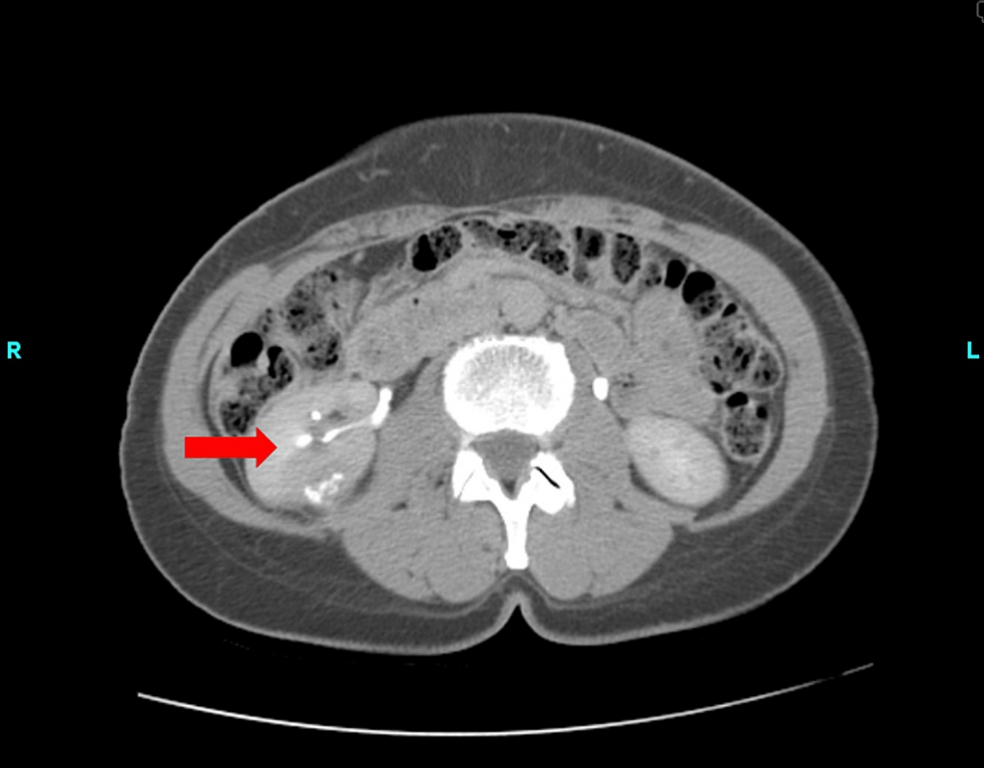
CT IV pyelogram CT IV pyelogram showing a complex cystic mass with calcifications of the right kidney (red arrow). CT IV: computed tomography intravenous
